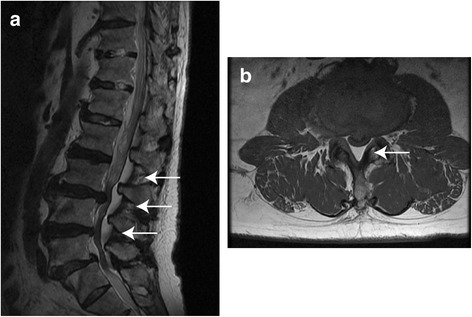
Magnetic resonance imaging T1 weighted view. a A sagittal scan shows hypertrophy of epidural fat predominately located posterior from L5 to S1 level. b A axial scan of L3-L4 revealed excessive amount of fat within the epidural space compressing the thecal sac. A high signal intensity lesion corresponds to fat (*white arrows*)
